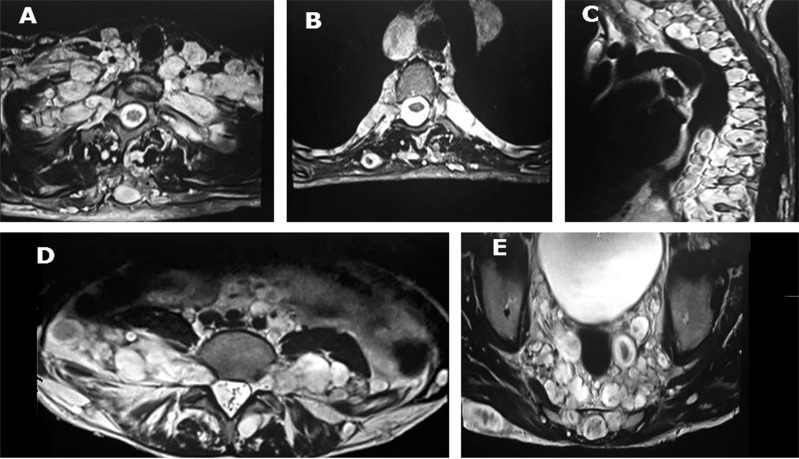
Widespread neurofibromas in neck, thorax, abdomen and pelvis; Axial T2-weighted image demonstrates widespread plexiform neurofibromas in the neck (A), Axial and parasagittal T2-weighted magnetic resonance imagings (MRI) demonstrate widespread cervical and thoracic paravertebral and mediastinal neurofibromas arising from cervicothoracic nerve roots (B and C), Axial T2-weighted MRI demonstrates multiple neurofibromas in the lumbar paravertebral, neural foraminal, para-aortic and retroperitoneal regions. Anterior displacement of bilateral psoas muscles is seen (D), Axial T2-weighted MRI demonstrate widespread pelvic neurofibromas in the perirectal and presacral spaces (E).
